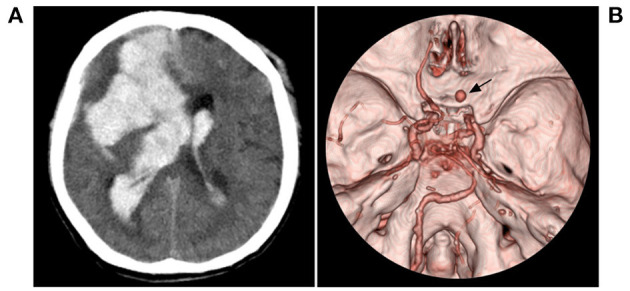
Fatal hemorrhage in case 5 in Table 2. (A) CT showed hemorrhage (arrow) in the right temporal lobe involving the ventricle. (B) CTA showed an aneurysm (arrow) in the right moyamoya vessels, and bilateral MMD can be seen. CT, computed tomography; CTA, computed tomography angiography; MMD, moyamoya disease.
